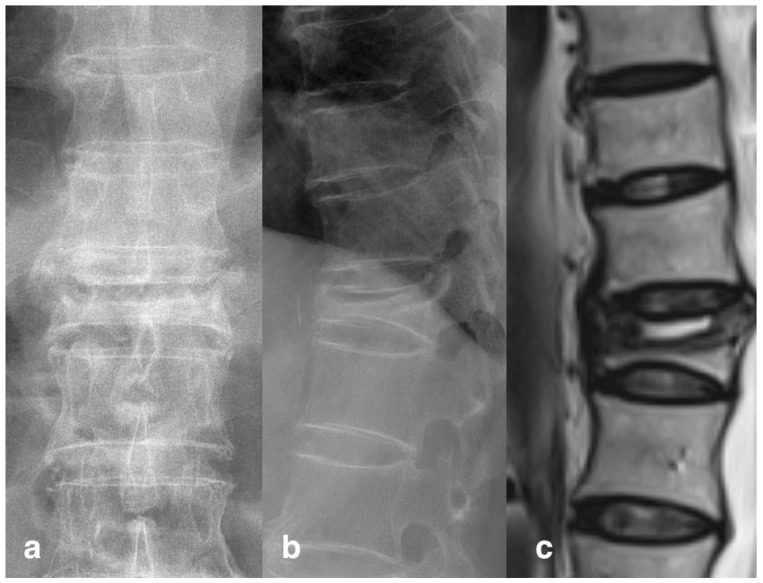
Vertebral cleft sign. The radiography of a patient with T12 compression fracture showed a typical cleft sign in (a) AP view and (b) lateral view. The sagittal view on T2 weight imaging MRI (c) also revealed a fluid-containing empty space in vertebral body.
